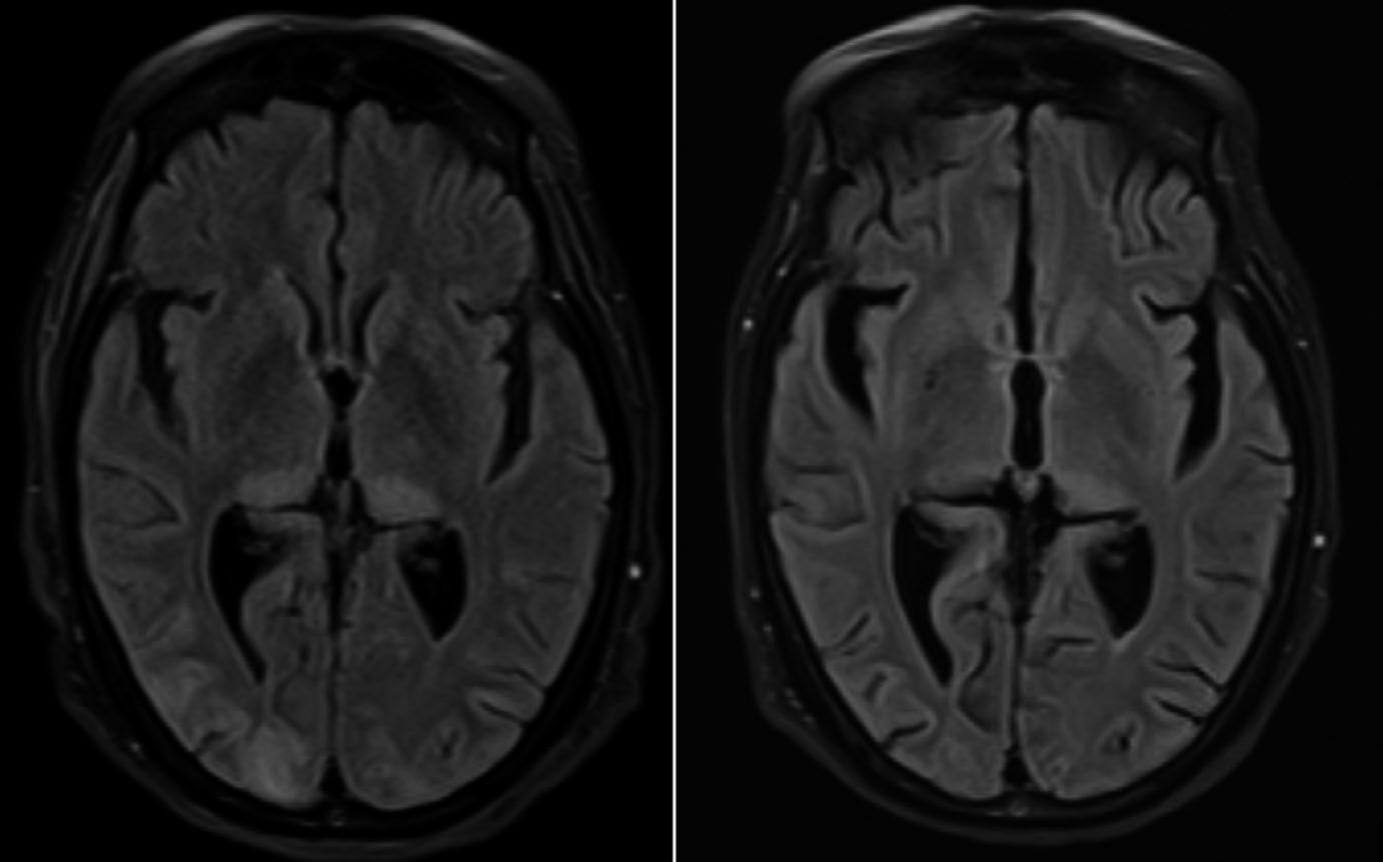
Fluid‐attenuated inversion recovery of MRI on day 2 of admission showing abnormal signal at bilateral pulvinar areas. On day 10 of admission, repeat MRI shows resolution. Also noted is the typical involvement of right occipital cortical and subcortical white matter in PRES
